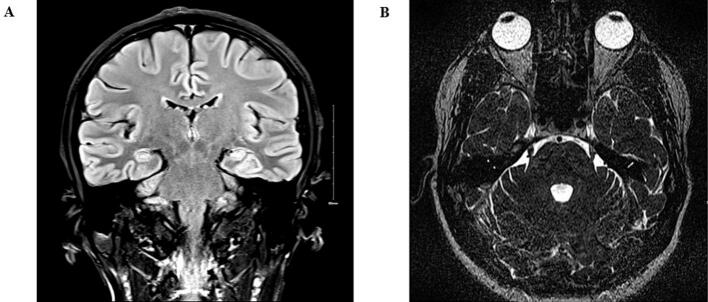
Examples of 3 T brain magnetic resonance imaging (MRI). A: T2 Coronal flair without an evident structural abnormality. B: T2-weighted axial did not reveal vascular compression of the trigeminal nerve.
